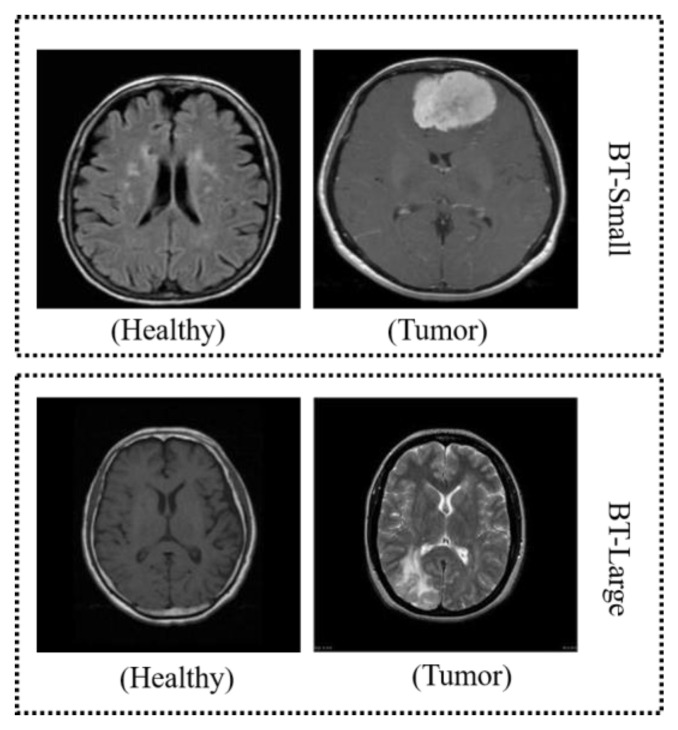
MRI samples from the employed datasets.
